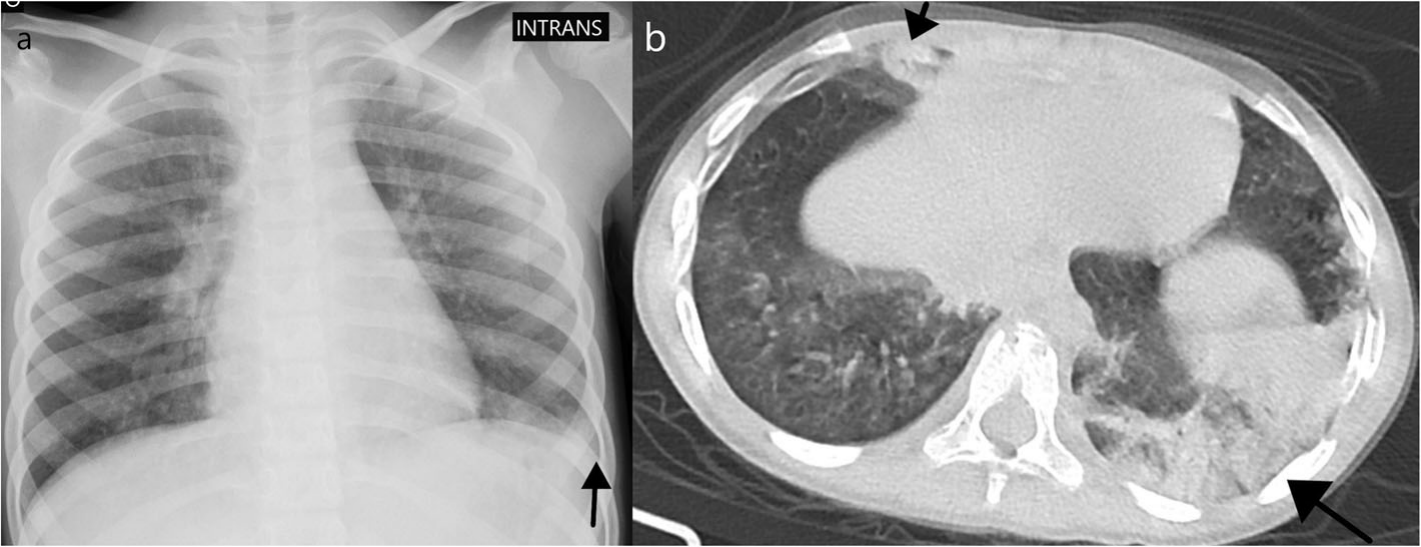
Indeterminate features in a 5-year-old asthmatic girl—CXR showed unilateral lower opacity (a), which is not typical for COVID-19. CT (b) was performed 6 days after the onset of symptoms, which showed bilateral peripheral consolidations, mainly in the left lower lobe but also in the middle lobe (arrows) and internal segment of the lower right lobe. The predominant pattern is consolidation, which is not typical for COVID-19, although a characteristic peripheral and lower distribution is seen
